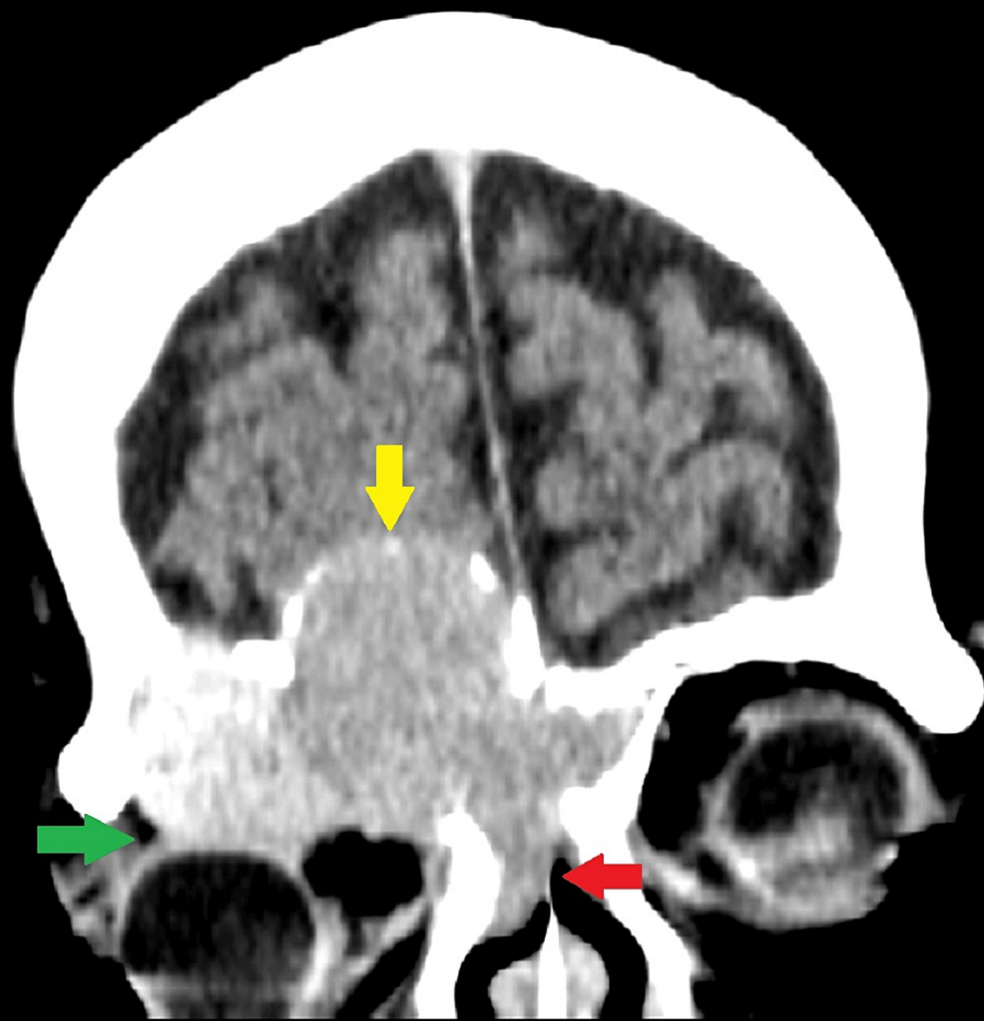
CT brain (plain) coronal view Large, heterogeneous, predominantly hyperdense mass in the right frontal sinus, destroying the walls of the right frontal sinus, resulting in compression of the right frontal lobe (yellow arrow). The mass also extends into the ethmoid sinus (red arrow) and the right orbit (green arrow), causing proptosis of the right eyeball.
